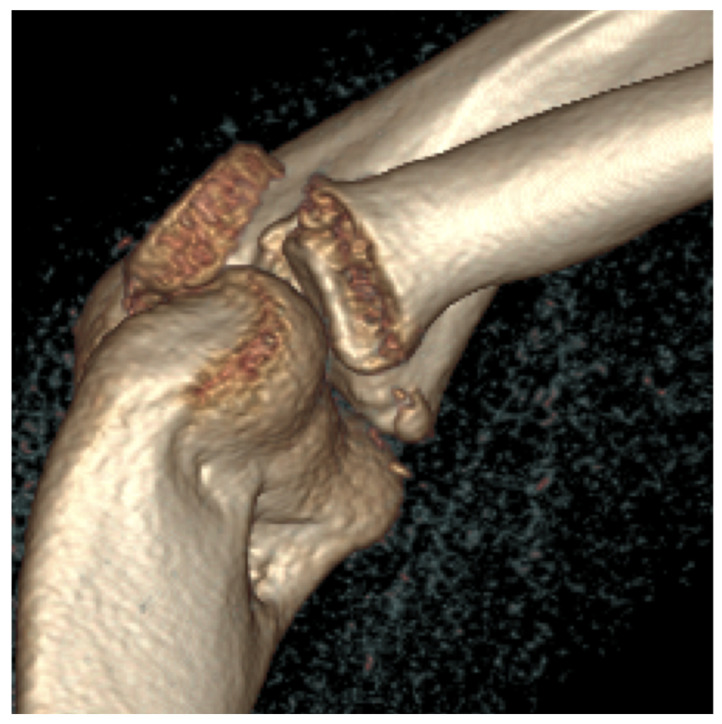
The CT images confirm the Mason type II fracture and dislocation of the radial head.
